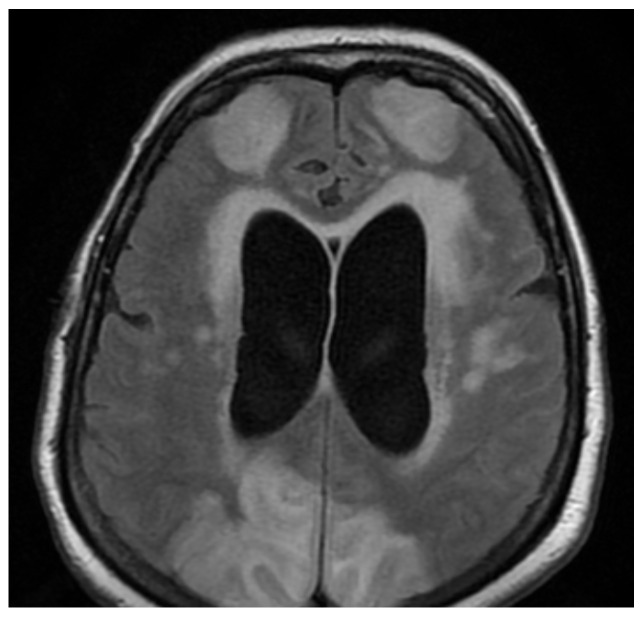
MRI axial fluid-attenuated inversion recovery (FLAIR) sequence noting bilateral subcortical hyperintense lesions, especially in the occipitoparietal lobes, resembling posterior reversible encephalopathy syndrome (PRES)-like findings.
